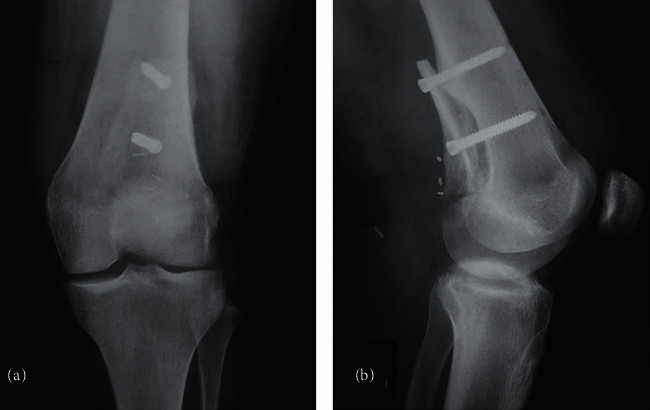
Anteroposterior (a) and lateral (b) radiographs 6 months postoperatively.
